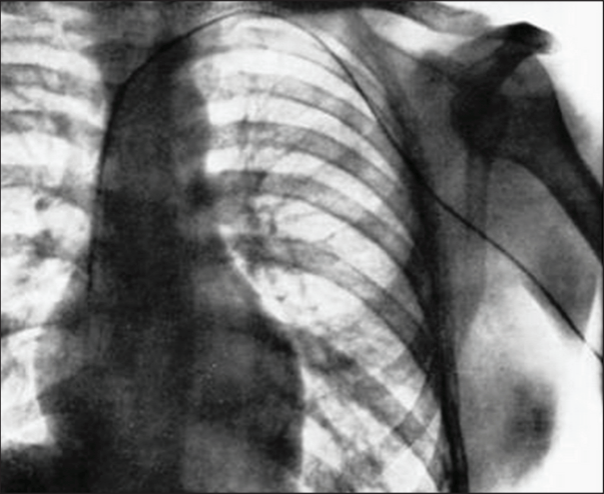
Radiography of the heart, catheterized by W. Forssman in Eberswalde (Germany) [5].
